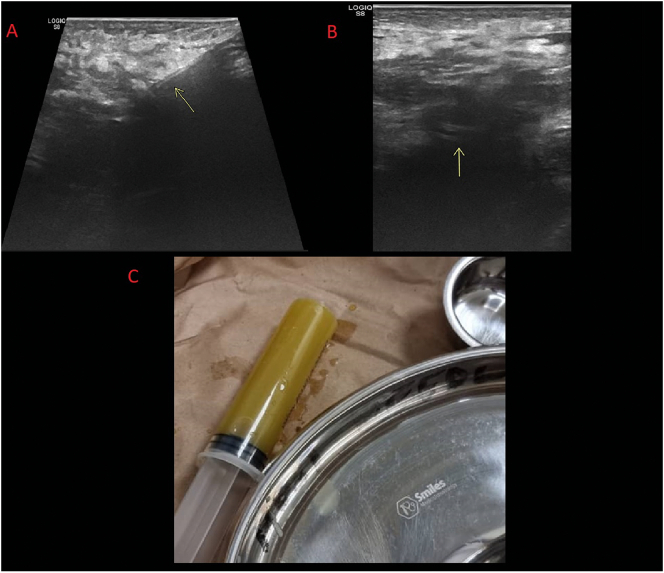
A to B – Ultrasound guided pigtail insertion into abscess. C – Initial pus aspirated.
